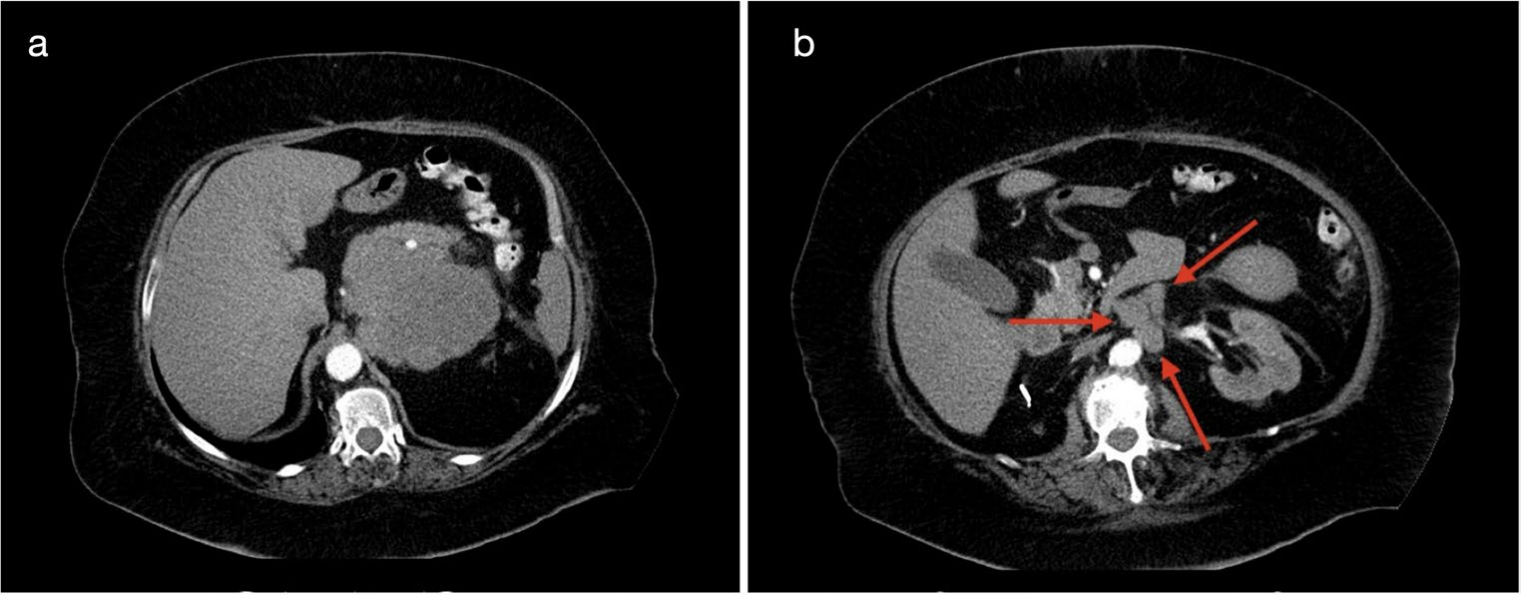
Computed tomography of a female patient with left-sided ACC a) primary tumor (9 cm), b) paraaortic lymph nodes metastases (arrows)
